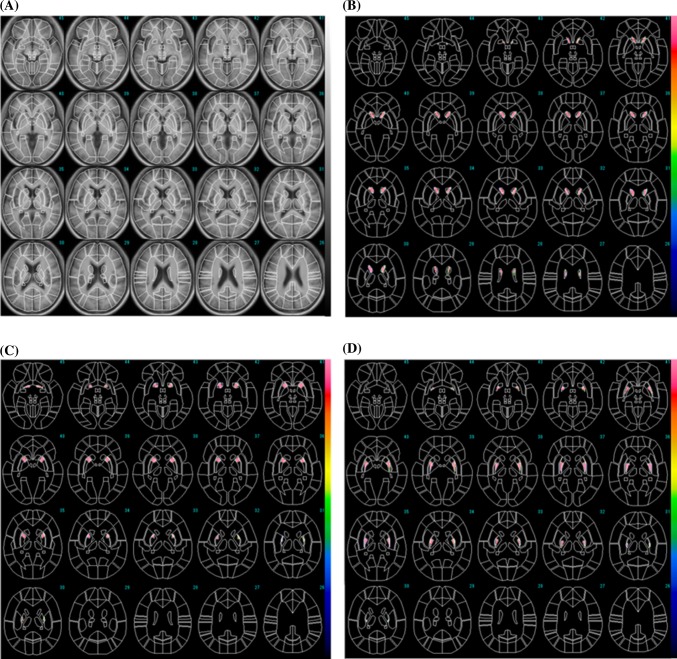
MRI T1-WI images and MRI-based ROIs were shown on the FineSRT system. a T1-WI images, b caudate ROIs, c anterior putamen ROIs, and d posterior putamen ROIs displayed using the FineSRT system
